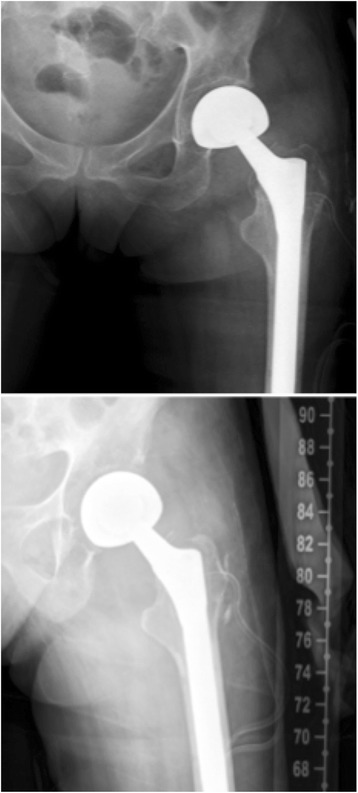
Post-operative (12 months) AP pelvic x-ray showing the bipolar hemiarthroplasty following removal of the cephalomedullary nail
